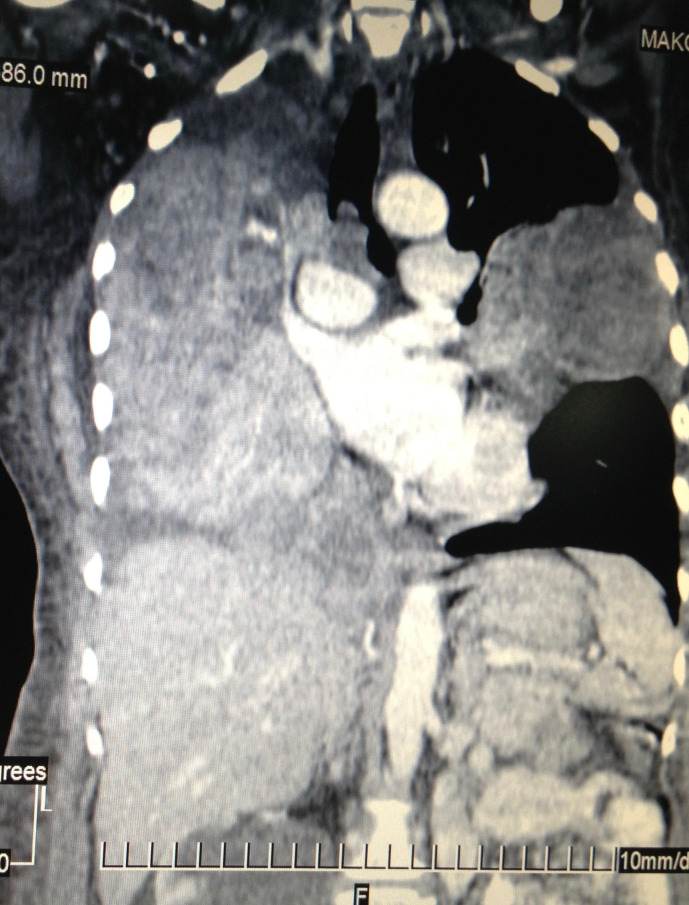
Coronal computed tomography scan showing bilateral
